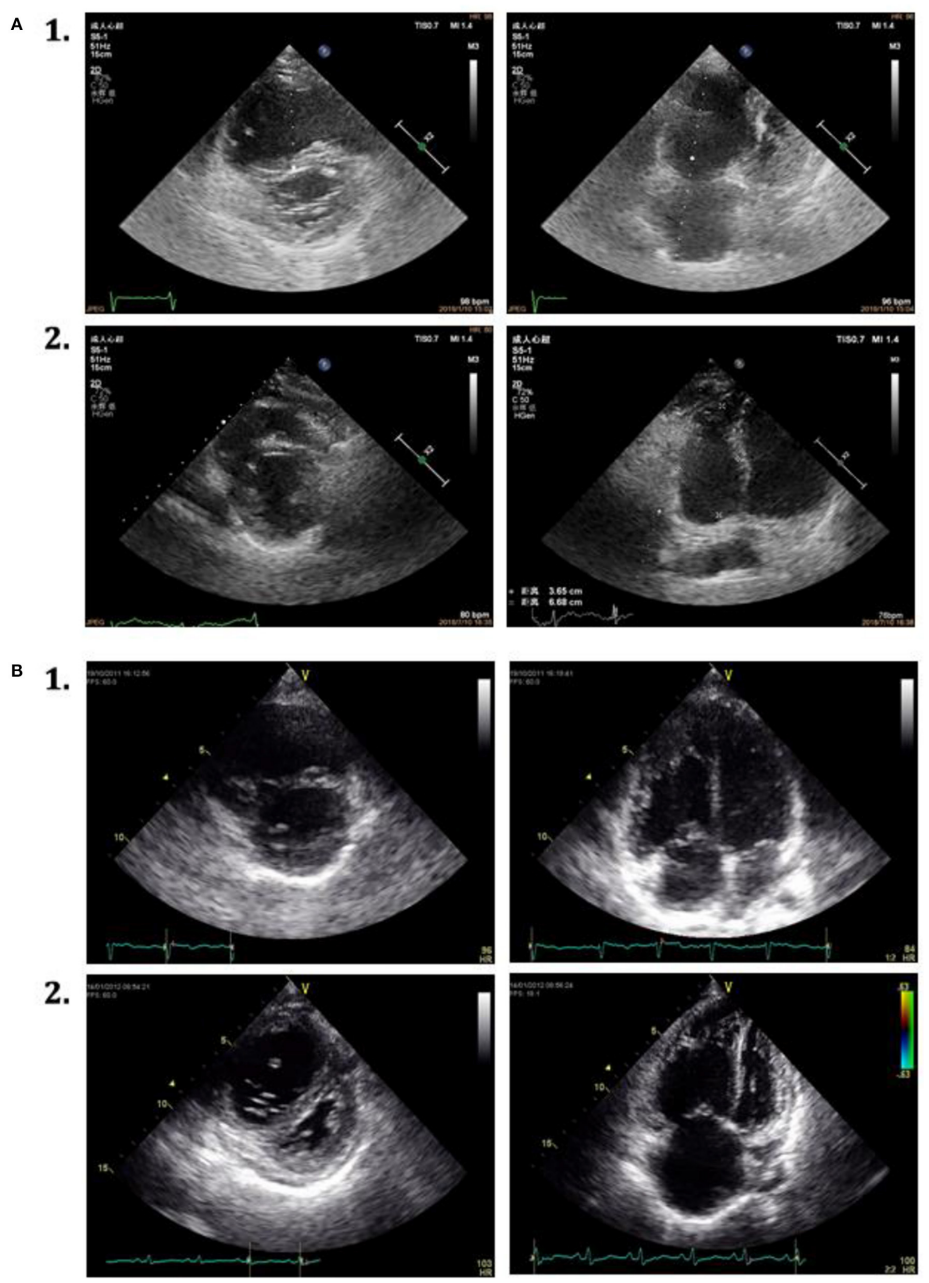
Echocardiographic parasternal short-axis view and apical four-chamber view in 2 patients with IPAH. (A) 1. The characteristics at baseline. 2. Attenuated right heart remodeling of the same patient at the first follow-up assessment. (B) 1. The characters at baseline. 2. Without attenuated right heart remodeling of the same patient at the first follow-up assessment.
